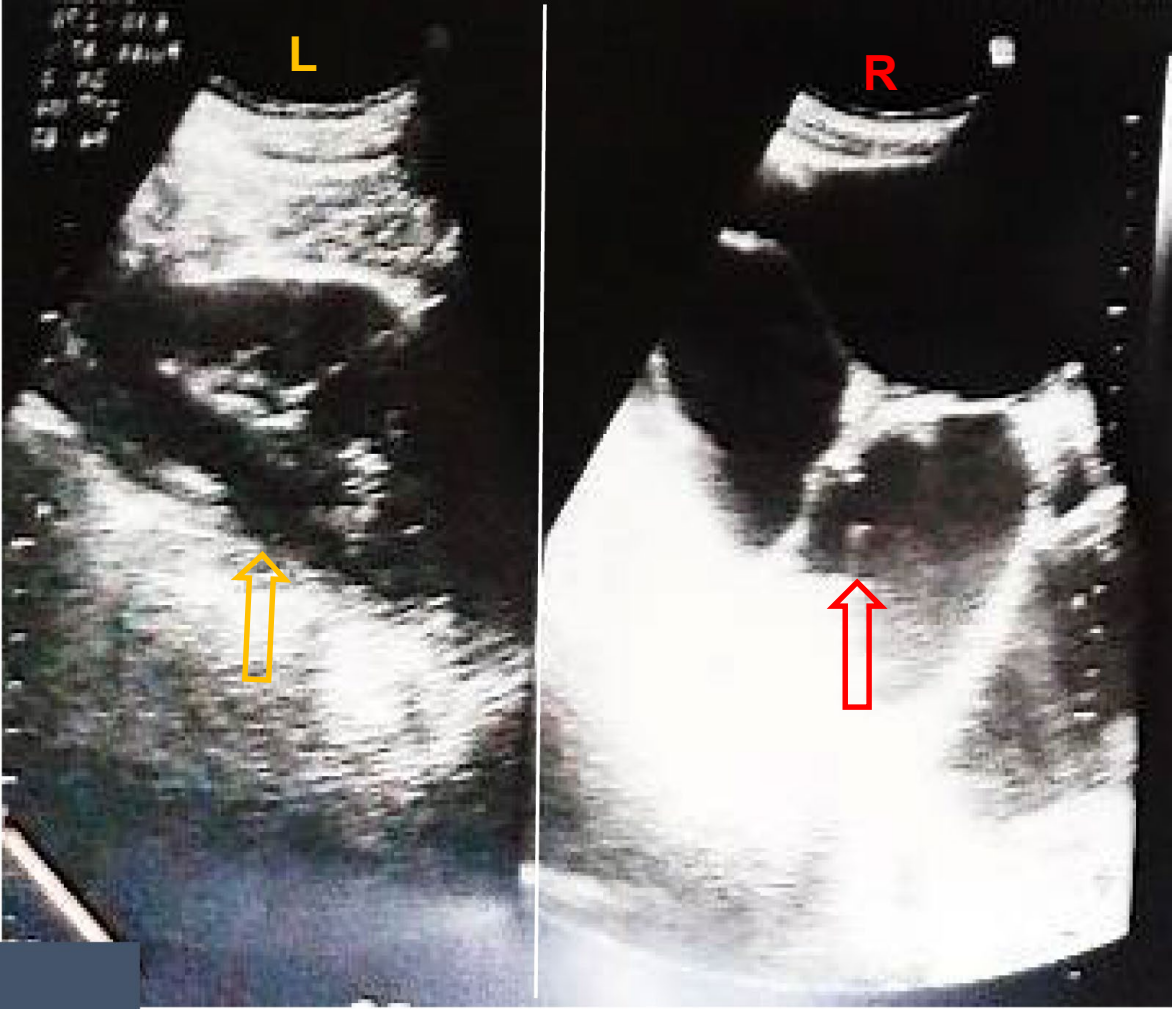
Abdominal ultrasound showing the left (L) and the right (R) kidneys. The left kidney is normal (*yellow arrow*) while the right kidney has multiple fluid filled cysts (*red arrow*)
